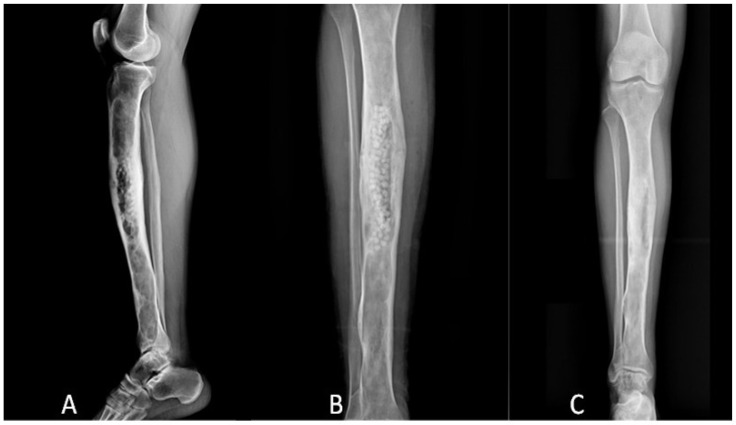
X-rays of the leg in a patient treated with debridement and adjuvant PerOssal bead for a chronic osteomyelitis of the tibia diaphysis. (A). Post-operative lateral view X-rays. Antero-posterior X-rays showing no filling at 6 months of follow-up (B) with complete filling at 12 months of follow up (C).
